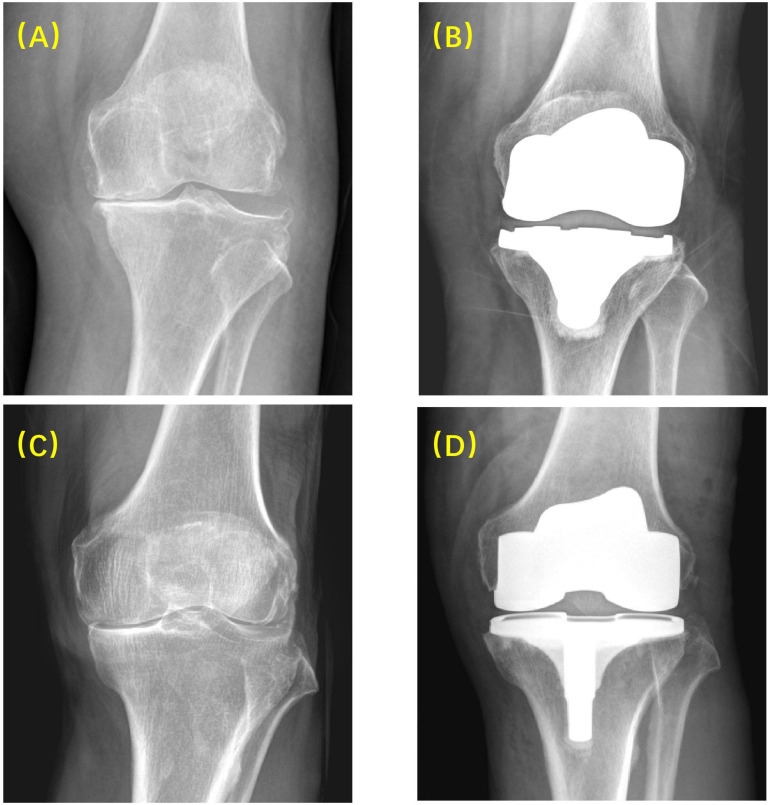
Radiographic images before and after surgery using MP and PS prostheses. (A) Before MP TKA; (B) after MP TKA; (C) before PS TKA; (D) after PS TKA.
